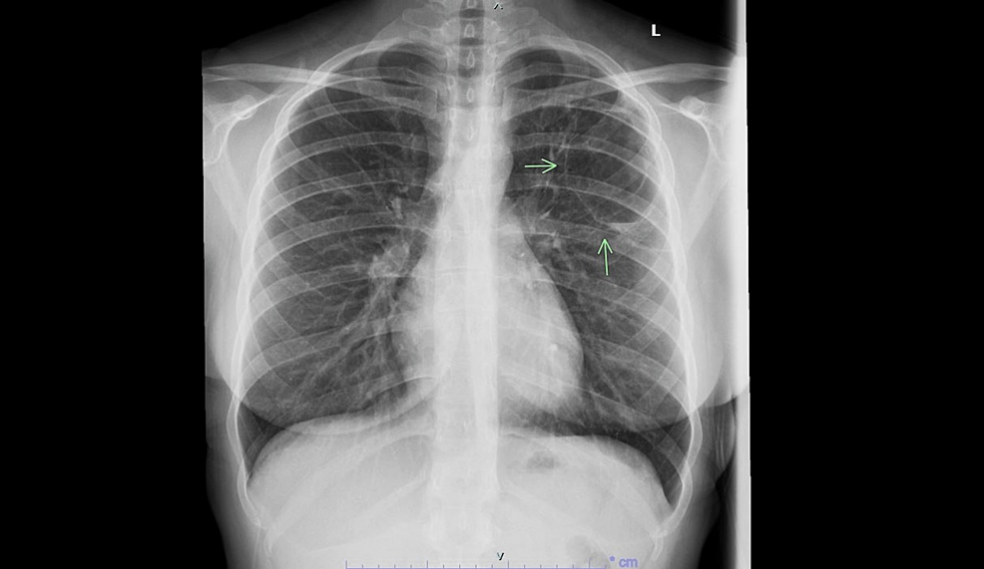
Chest X-ray done three weeks after antibiotics showing resolution of air fluid levels and improvement in the left lung abscess
